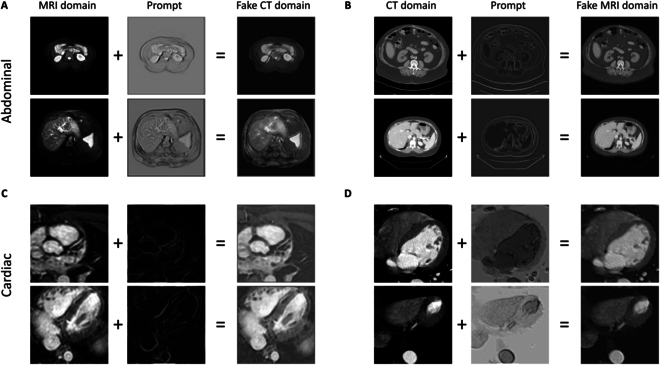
Visualization of alignment prompt. (A and B) Samples of abdominal dataset. (C and D) Samples of cardiac dataset. (A and C) CT→MRI adaptation task. (B and D) MRI→CT adaptation task.
